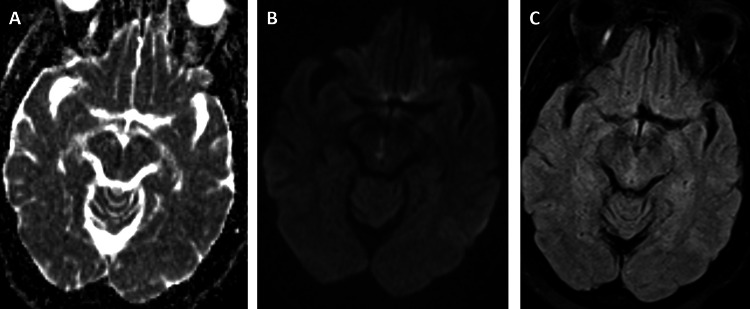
Case 1 MRI Axial MRI images including (A) ADC, (B) DWI, and (C) FLAIR.  Images demonstrate restricted diffusion with associated FLAIR signal abnormality in the right paramedian midbrain. ADC, apparent diffusion coefficient; DWI, diffusion-weighted imaging; FLAIR, fluid-attenuated inversion recovery.
